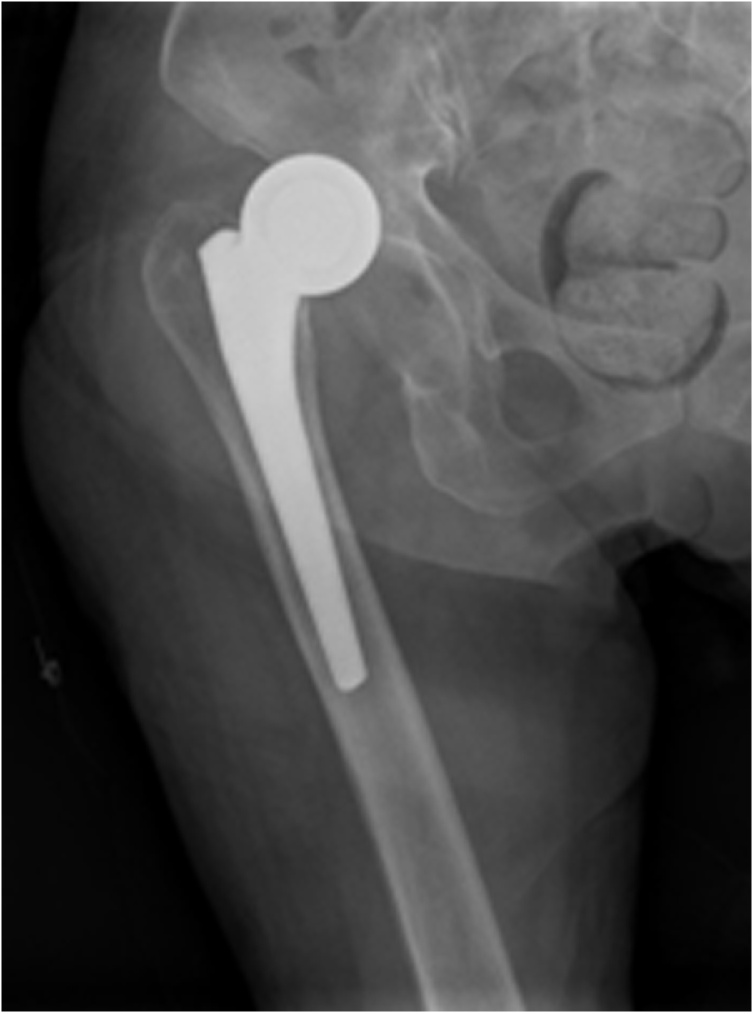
Anteroposterior radiograph of the right hipdemonstrating a dislocation of right hip hemiarthroplasty.
